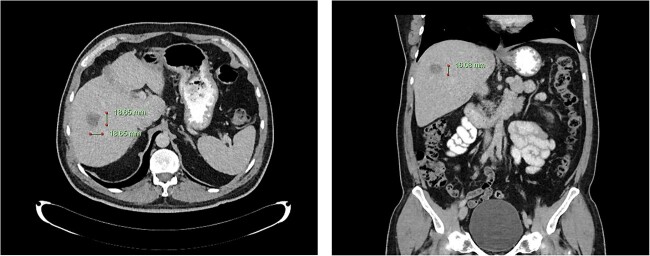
Computed tomography dated 25 September 2012 of the abdomen and pelvis measuring 18.65 mm (anterior–posterior) lesion at liver segment 5/6 in axial (left) and coronal (right) view.
